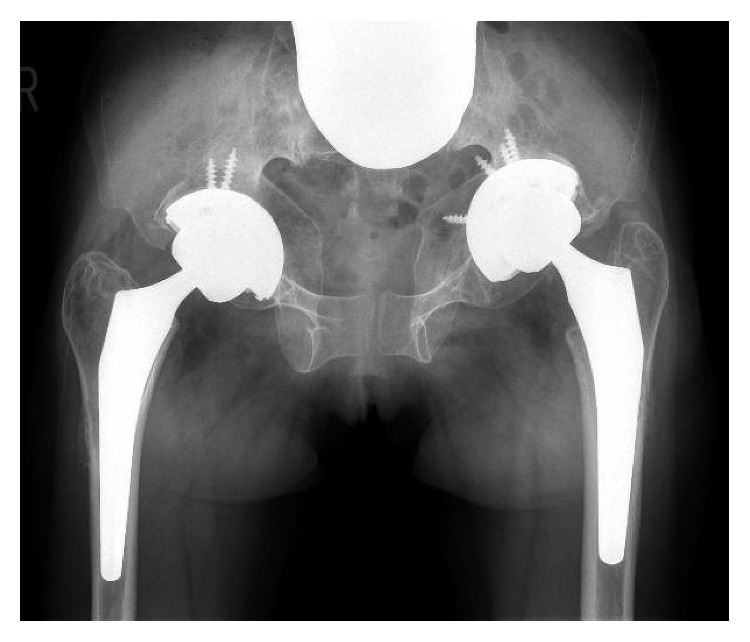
Postoperative radiograph of the hip joints, taken 1.5 years after the last surgery, demonstrating well-fixed components.
